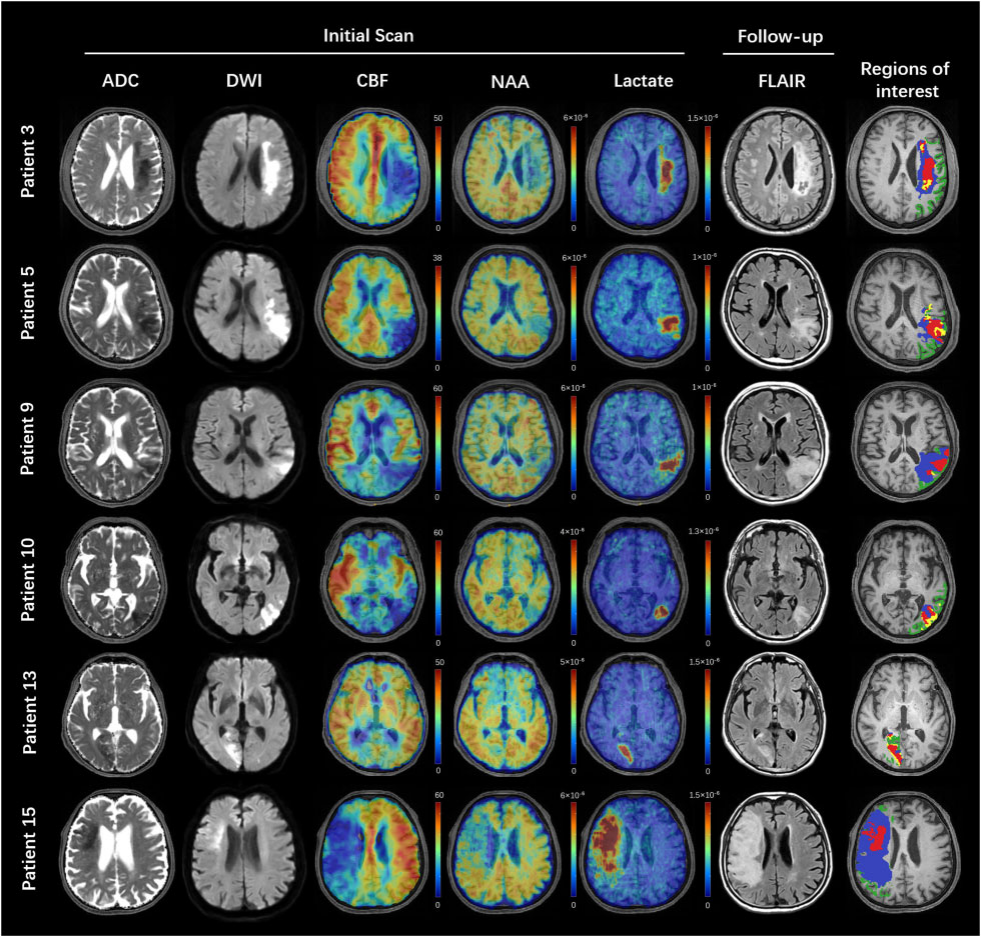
Multimodal images from representative patients. All the images were registered to the structural T_1_-weighted images. The ADC, DWI, cerebral blood flow and MRSI images were acquired in the first scan. The FLAIR images were acquired in the follow-up session. The colour bar for ASL-PWI shows the cerebral blood flow in ml/100 g/min. The colour bar for MRSI shows NAA or lactate level in institutional units. Regions of interest: light green = oligemia; blue = infarct growth; yellow = DWI reversal; red = infarct core.
